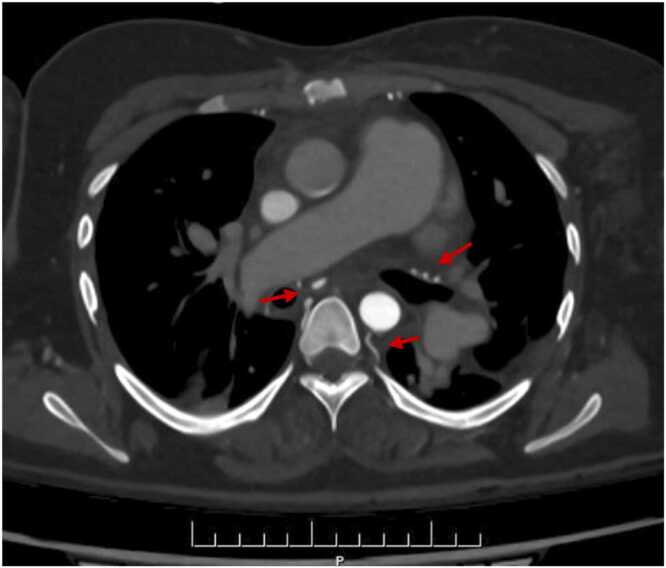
Computed tomography angiogram of the chest showing hypertrophic systemic bronchial and nonbronchial arteries in a patient with Eisenmenger syndrome complicating an uncorrected ventricular septal defect.
